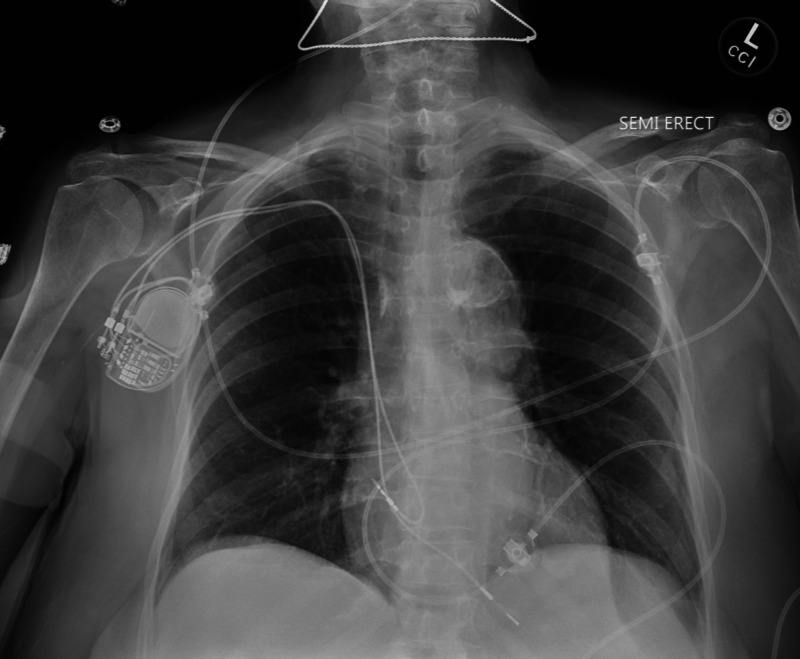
Chest x-ray without evidence of acute cardiopulmonary abnormality. Note image without evidence of significant pulmonary edema
